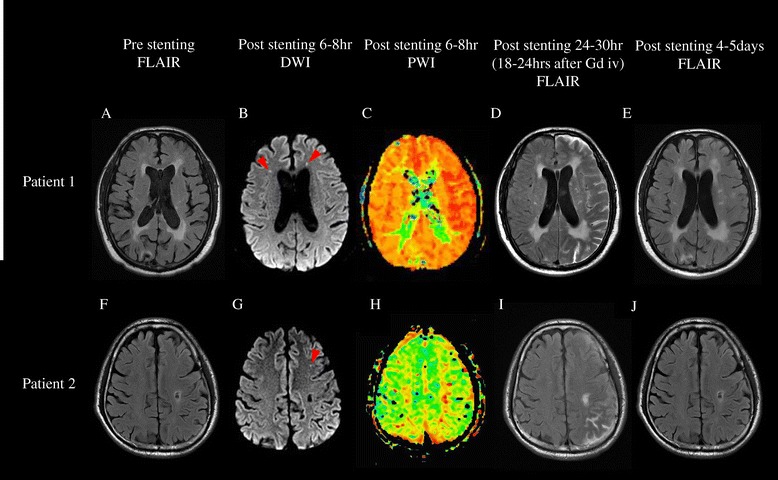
Serial follow-up FLAIR MRI. Chronic ischemic white matter changes were observed in pre-stenting FLAIR MRI (A and F). Post-stenting FLAIR MRI performed 18–24 hours after intravenous Gd showed hyperintensities in the subarachnoid space (D and I), which resolved in 4–5 days (E and J). Post-stenting DWI showed a few small subcortical lesions (arrowheads in B and G). Slightly increased perfusion on the time-to-peak map in the left hemisphere was observed in patient 1 (C).
